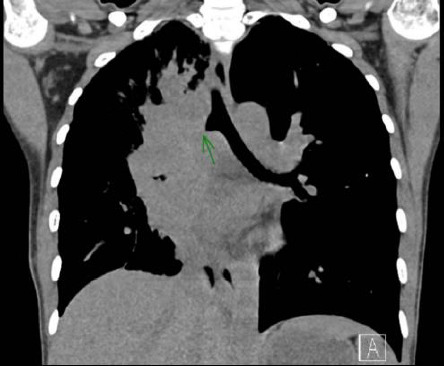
Chest CT. Chest CT showed extensive matted lymph nodes in the right hilar, paratracheal, subcarinal, interlobar, right supraclavicular, and right lower cervical regions with extensive peribronchovascular soft tissues, causing total right mainstem bronchial obstruction, obliteration of the segmental bronchi of RUL and RML.
